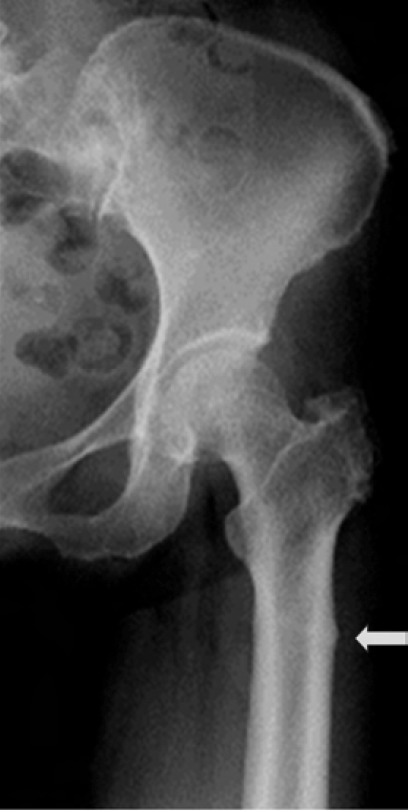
Radiograph of left hip showing a
